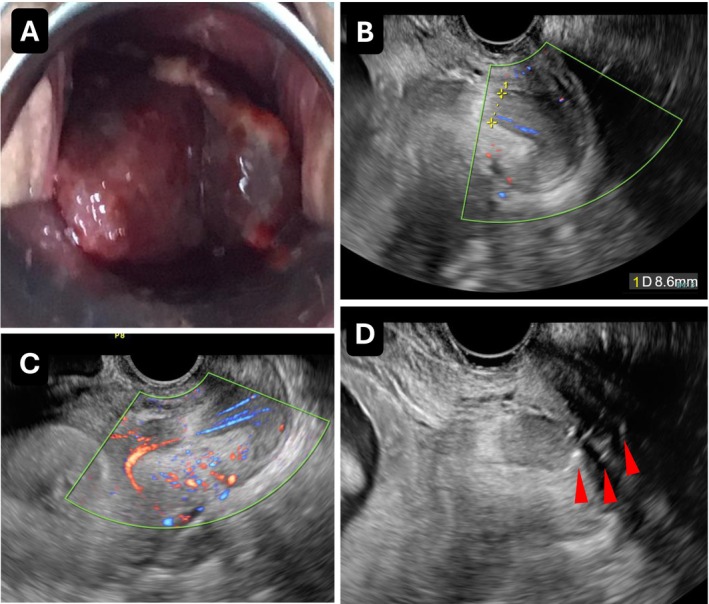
Initial presentation and ligation of a giant cervical polyp. (A) A giant cervical polyp measuring approximately 4 cm in diameter, occupying the vagina and obscuring the portio vaginalis uteri at 11 weeks and 5 days of gestation. (B) Transvaginal ultrasound revealing a thick polyp stalk measuring 8.6 mm in diameter. (C) Color Doppler ultrasound demonstrating blood flow within the polyp stalk. (D) Ligation of the polyp root near the external cervical os using triclosan‐coated polydioxanone sutures (three ligations; arrow).
